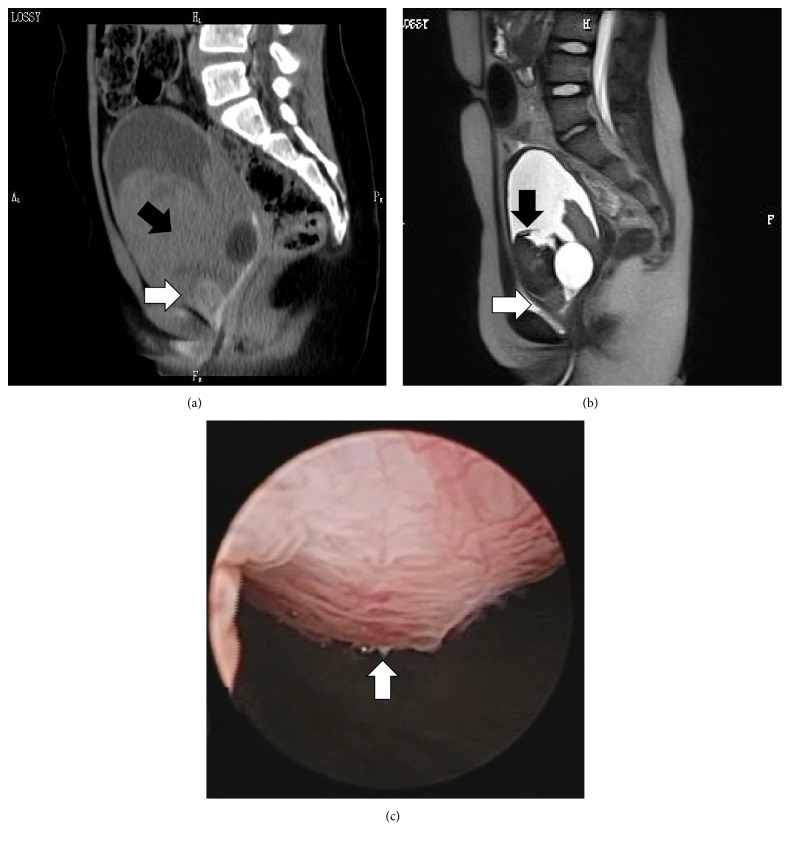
(a) A high-density lesion (sized 10 mm) is observed in the bladder neck on plain computed tomography images (indicated by a white arrow). A hematoma in the bladder is also observed (indicated by a black arrow). (b) A high-intensity lesion (sized 10 mm) is observed in the bladder neck on plain magnetic resonance T2-weighted images (indicated by a white arrow). A hematoma in the bladder is also observed (indicated by a black arrow). (c) A sessile nodular tumor with a smooth surface (sized 10 mm) located in the direction of 10 o'clock in the bladder neck is observed with cystoscopy (indicated by a white arrow).
